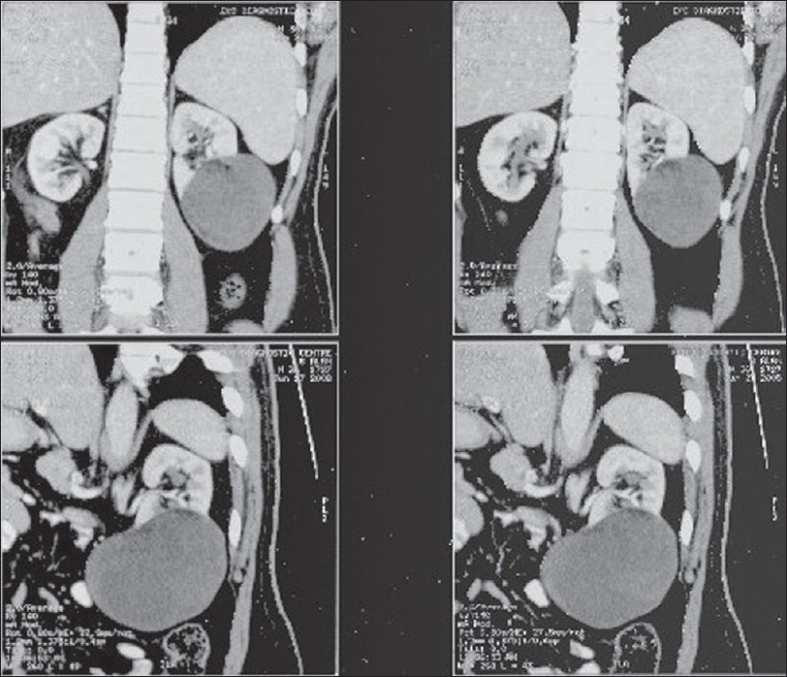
Contrast enhanced CT scan showing well-defined homogenously hypodense mass arising from the lower pole of the left kidney
